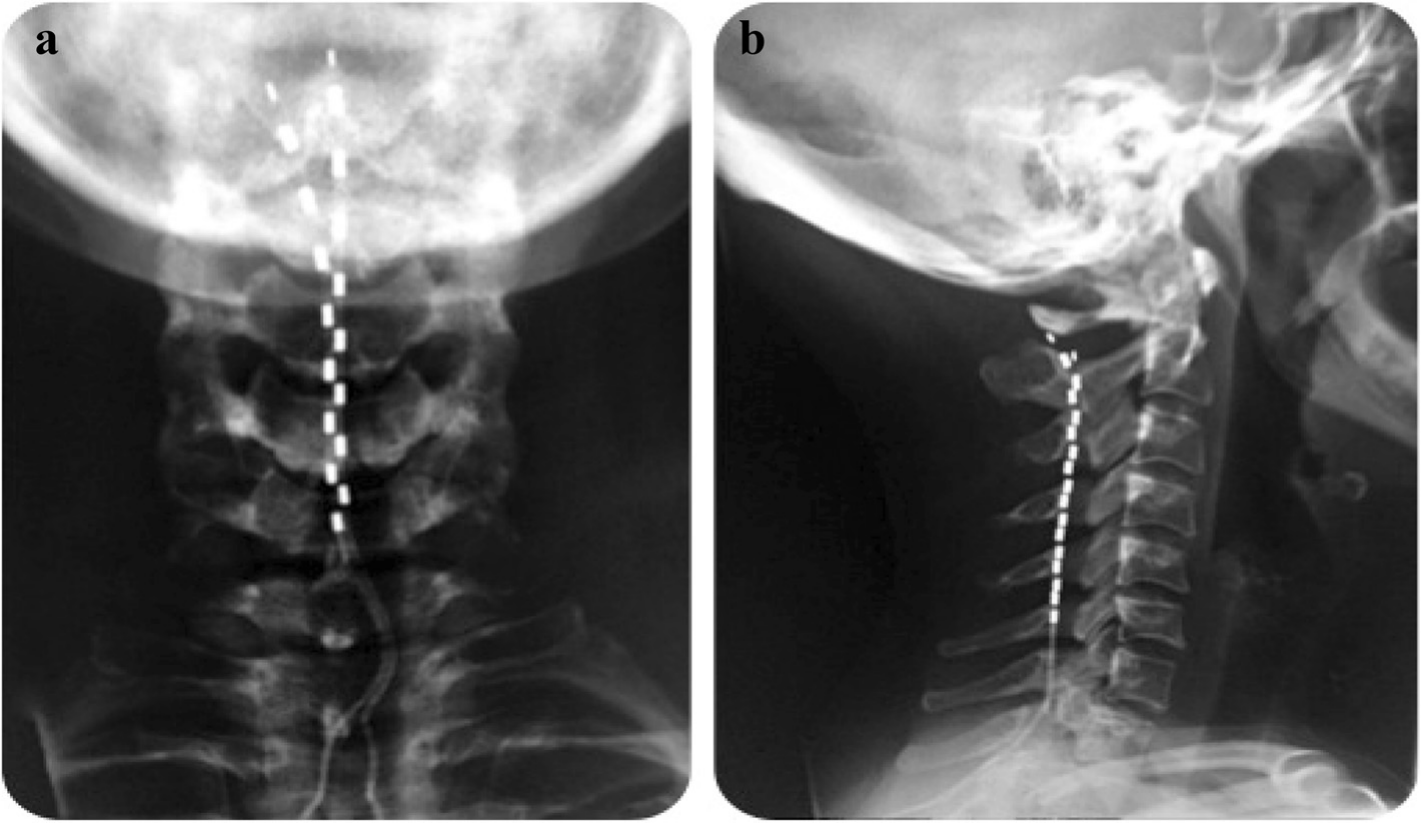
Antero-posterior (a) and lateral (b) radiographic view of the cervical spine to demonstrate the final position of the implanted leads within the posterior epidural space (SUNA patient, 42 months follow-up)
